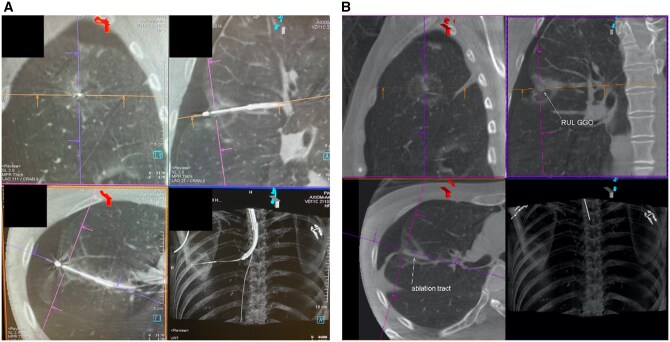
Patient D intraoperative CBCT. (A) Intraoperative fluoroscopy detected the shift in position of the ablation catheter. CBCT showed the catheter had shifted due to significant tissue contraction, resulting in protrusion into the pleural cavity. (B) Fibrin glue was injected along the ablation tract through the EWC, successfully sealing off the BPF. Abbreviations: BPF, bronchopleural fistula; CBCT, cone-beam CT; EWC, extended working channel.
